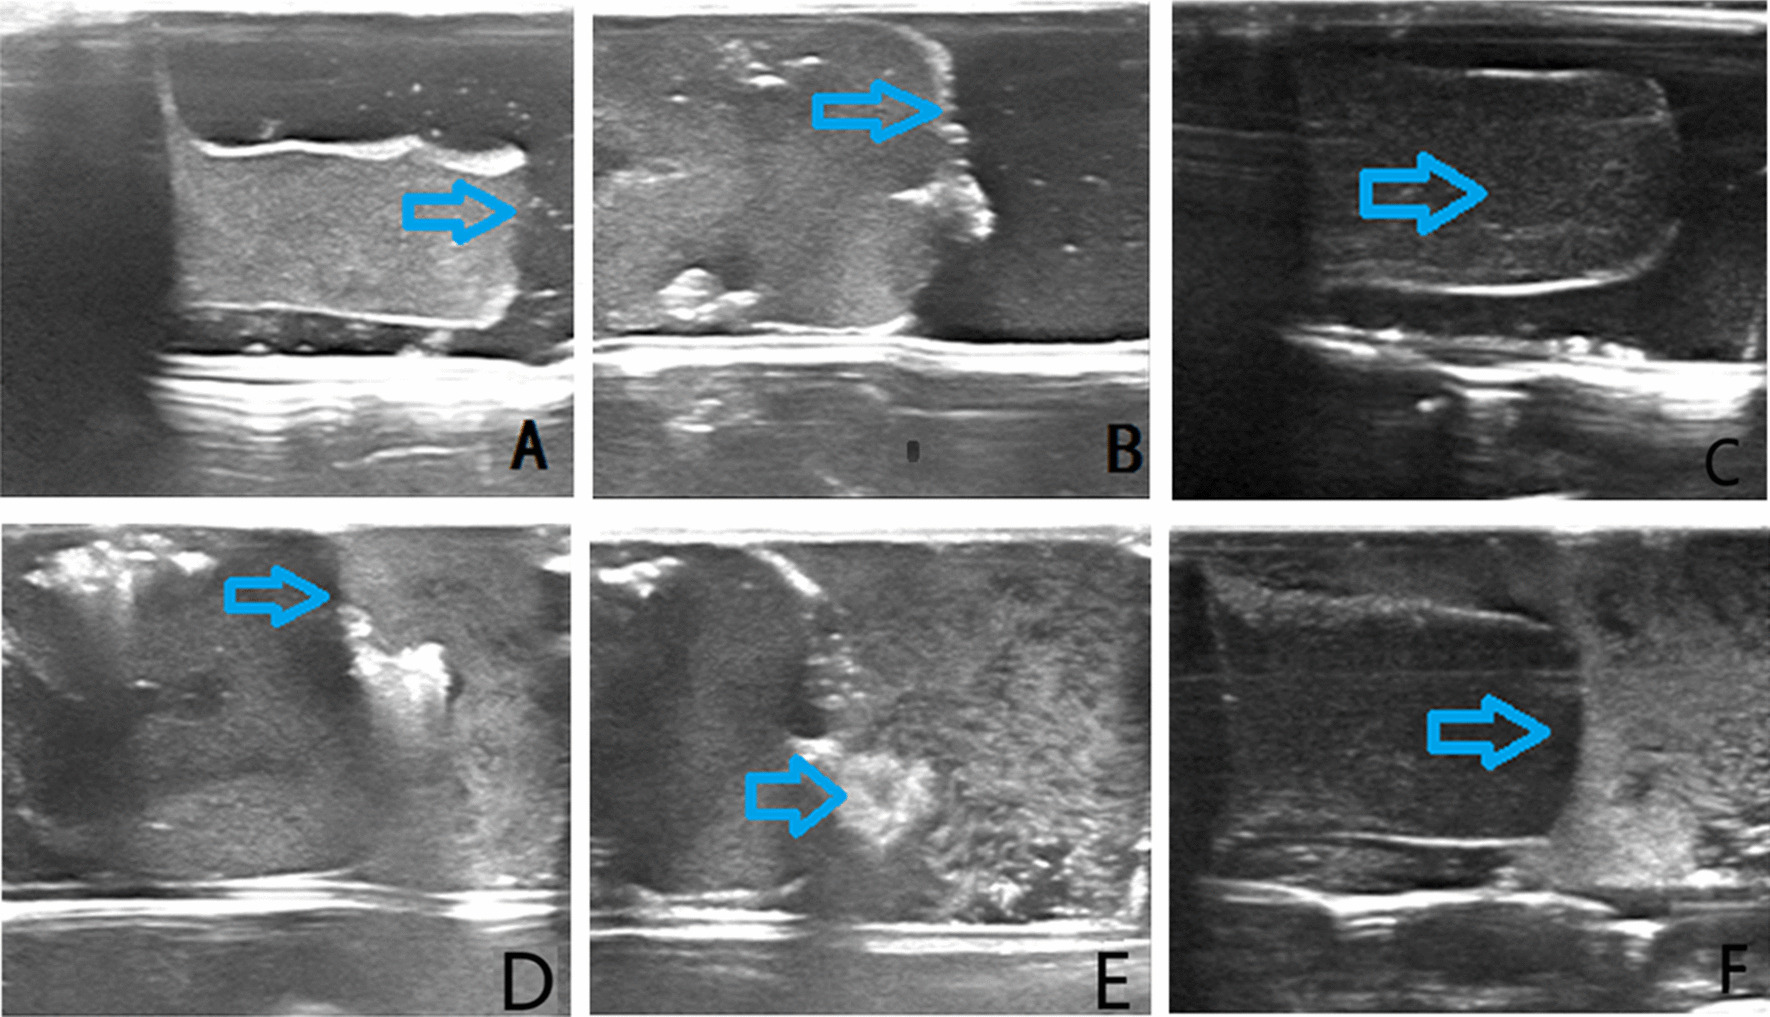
Two-dimensional conventional and contrast-enhanced ultrasound imaging of the thrombus at 1 h, 2 h and 7 days. a 1 h after thrombus formation, the surface of the thrombus was isoechoic (blue arrow). b 2 h after thrombus formation, volume increased and hyperechoic surface augmented (blue arrow). c 7 days after thrombus formed, uniformly hypoechoic core and slightly stronger surface echo (blue arrow). d 1 h after thrombus formation, ultrasound contrast enters freshly formed part of thrombus at surface (blue arrow), inconsistent with standard 2-dimensional morphology. e 2 h after thrombus formation, small thrombus (blue arrow) with obvious mobility on the surface of the thrombus, not shown by two-dimensional imaging. f No surface entry by contrast in thrombus of 7 days (blue arrow)
